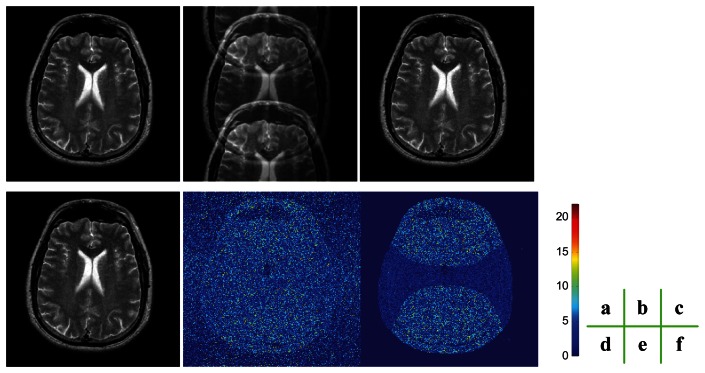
Comparison of the quality of the reconstructions: (a) Original, fully sampled T_2_-weighted brain MR image; (b) The aliased image; (c) Reconstructed image by ROS-based correction; (d) Reconstructed image by ROS-based reconstruction; (e) Difference between (c) and (a); (f) Difference between (d) and (a).
